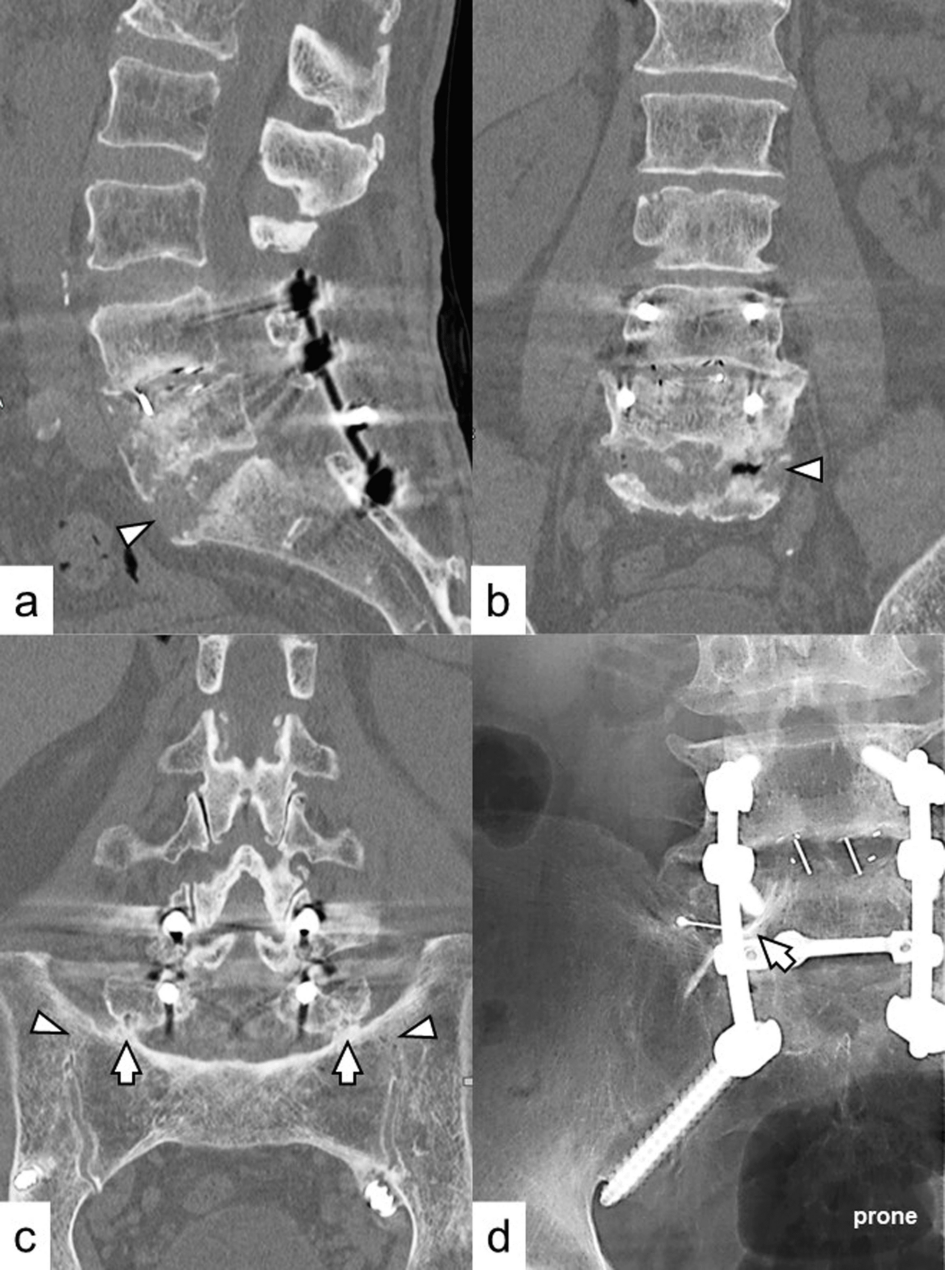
Imaging findings before surgery at our institute. According to the sagittal (a) and coronal (b) CT scans, bony fusion is not yet achieved at the L5-S1 intervertebral space, where the infected interbody cage has been removed (arrowheads). (c) Bony fusion between the transverse process of L5 and sacral alar (arrows) is evident bilaterally on CT. Furthermore, the bilateral sacroiliac joints have fused spontaneously (arrowheads). (d) The contrast medium in the left L5 diagnostic selective radiculography indicates that the left L5 nerve root is not compressed in the L5-S1 foraminal area (arrow), and no foraminal stenosis at L5-S1 is detected. CT, computed tomography
